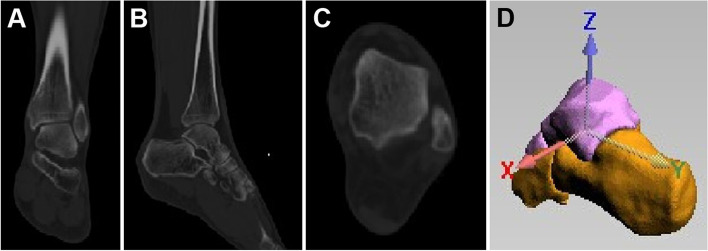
CT image of the ankle joint in coronal (A), sagittal (B) and transverse (C) views. (D) Setting of talus coordinate axis
